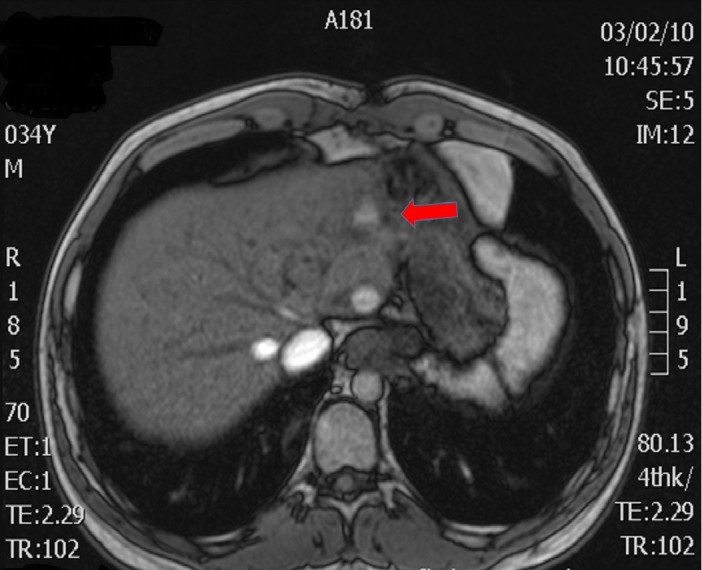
CT and MRI Preoperative Images Axial MRI - Attachment to left liver (arrow)
